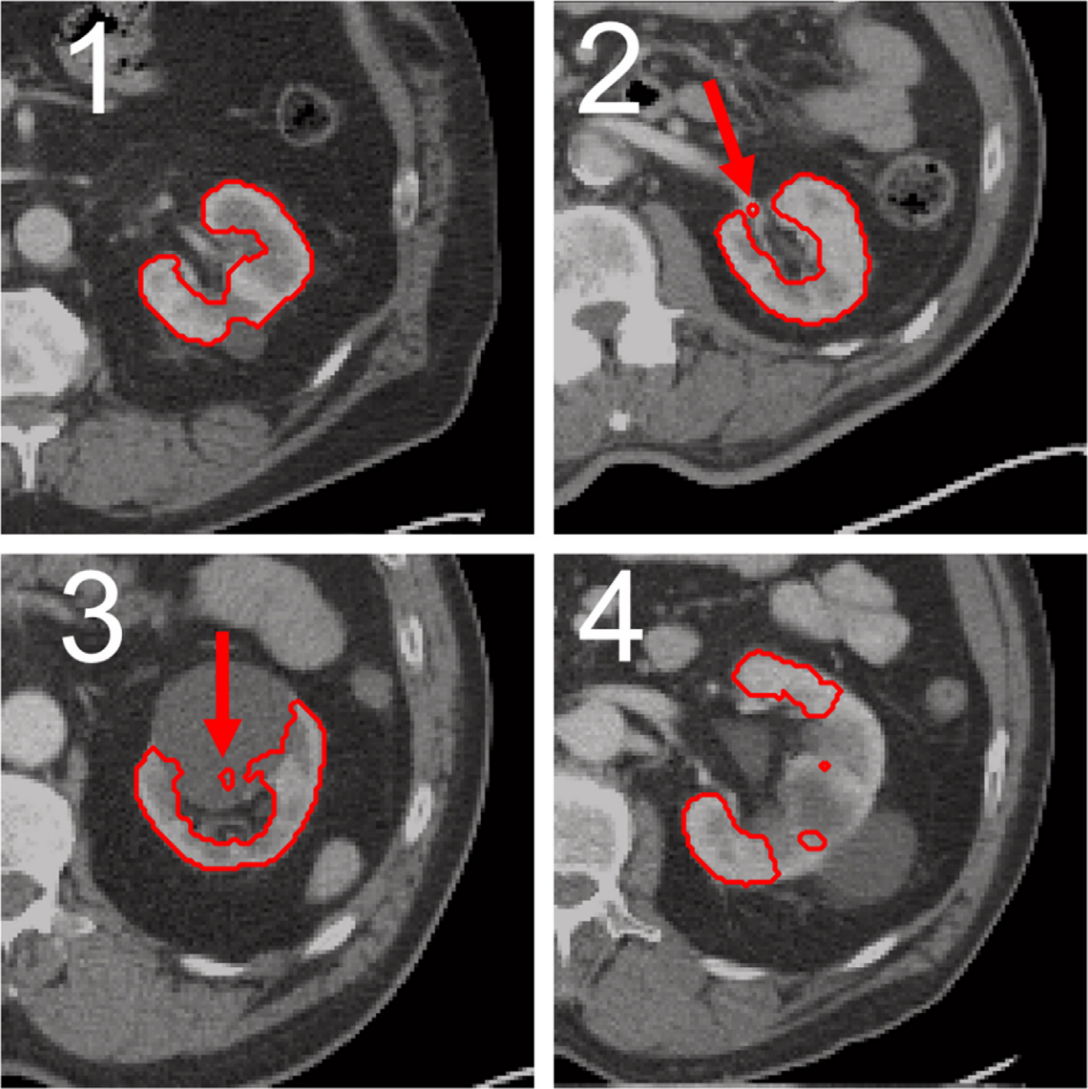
Exemplary representation of automatically generated segmentation of the four scoring values. All segmentations were created solely based on CT using approach A1. For the segmentation, which was rated with a score of 1 (upper left, image 1), no errors are recognizable. For the kidney on the top right (image 2) a small part of the renal hilus was segmented incorrectly (indicated by the red arrow), resulting in a score of 2. Bottom left (image 3) a segmentation containing parts of a cyst is shown (indicated by red arrow), resulting in a score of 3. For the segmentation (lower right, image 4), which was rated with a score of 4, rough errors are visible, as large parts of the kidney are not segmented.
